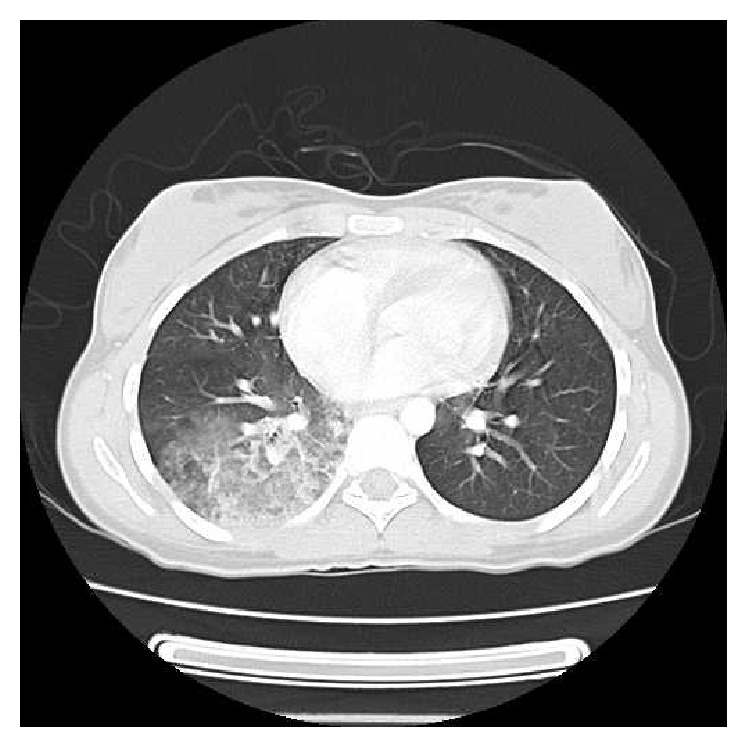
Spiral CT scan of the chest demonstrates an area of consolidation and surrounding ground glass opacity in the right lower lobe.
